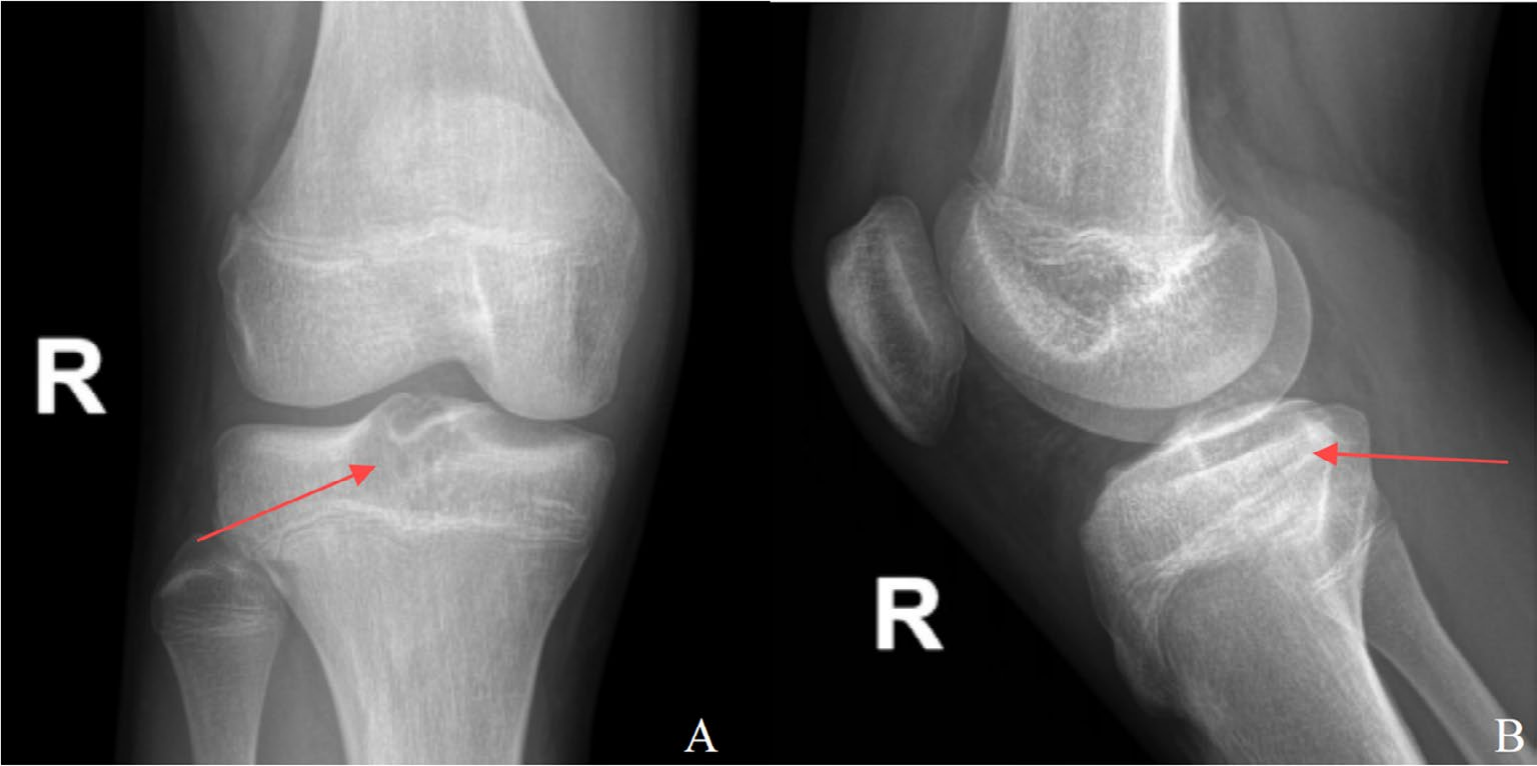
Radiographs of right (R) knee showing eccentric juxta‐articular osteolytic lesion in proximal tibial: Anteroposterior (A) and lateral (B) views.
